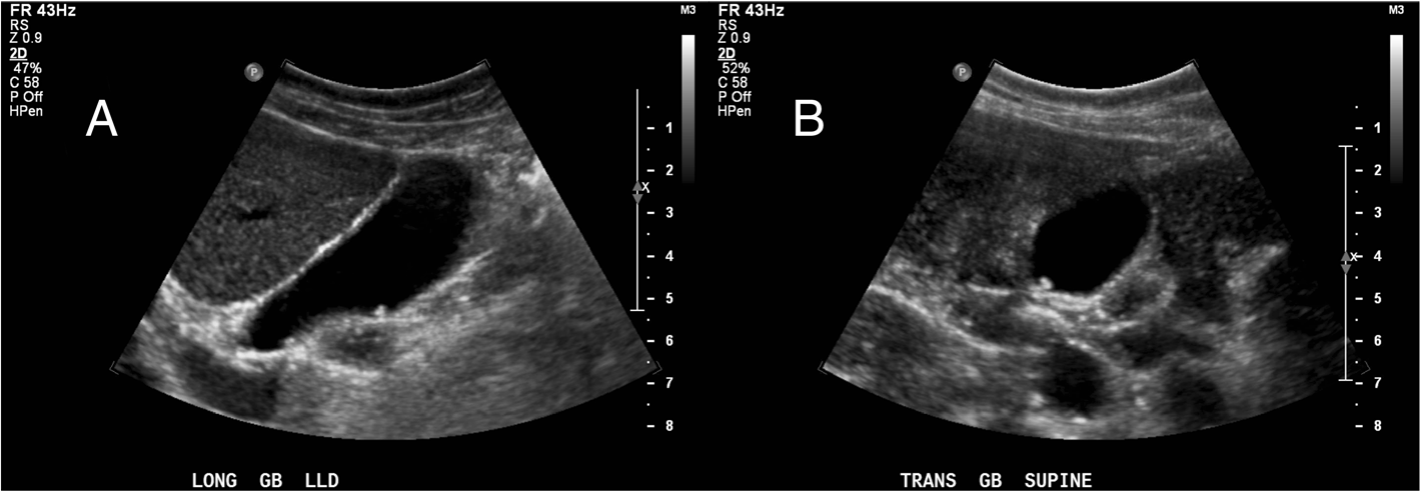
Formal right upper quadrant ultrasound #1. (A) Long and (B) short axis views showing a few small stones within the gallbladder, with no gallbladder wall thickening or pericholecystic fluid.
